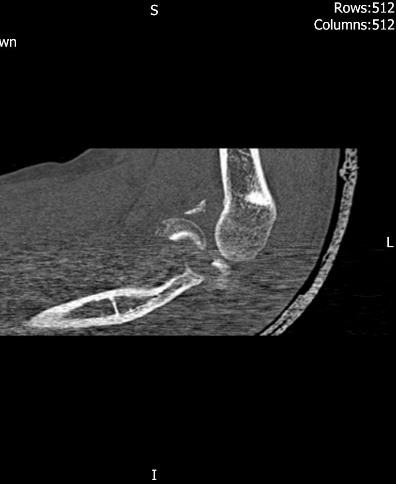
Pre-operative CT images of type IV injury.
